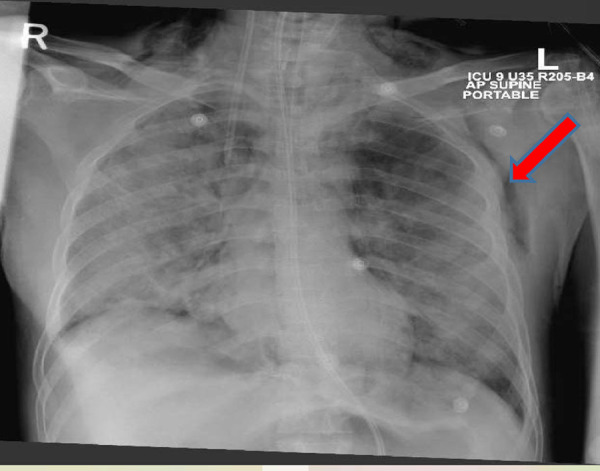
Chest X-ray image showing subcutaneous emphysema
